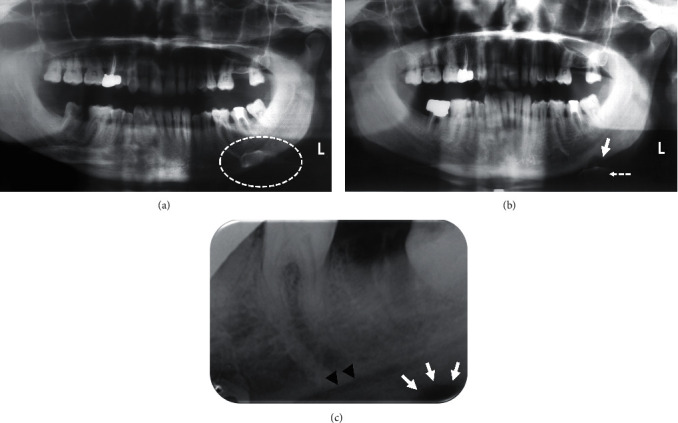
Standard radiological assessment. (a) Initial panoramic radiograph showing superposition of the hyoid bone on the left mandibular basilar border (white dashed circle), masking the osteolytic image. (b) Panoramic radiograph repeated with the patient in a slightly hyperextended position, allowing a lower projection of the hyoid bone (dotted white arrow), and a good visualization of the osteolytic image of the left basilar border (white arrow). (c) Retroalveolar radiograph performed after extraction of the tooth #37 showing the healing alveolus of this tooth and an osteolytic image (white arrows) below the mandibular canal (black arrowheads) at the lower limit of the radiograph.
